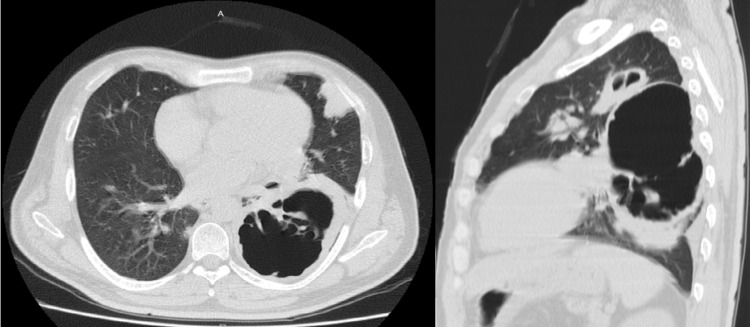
Chest computed tomography scan. A chest computed tomography scan in transverse (A) and sagittal views (B) showed left lower lung cavitation measuring 86 mm in diameter.
